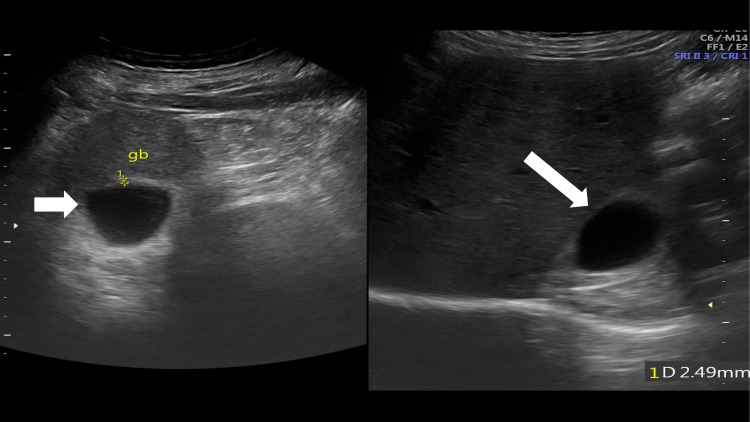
Ultrasound gray-scale images show an anechoic well-defined cystic structure in the posterior-inferior aspect of the liver, consistent with the gallbladder.
